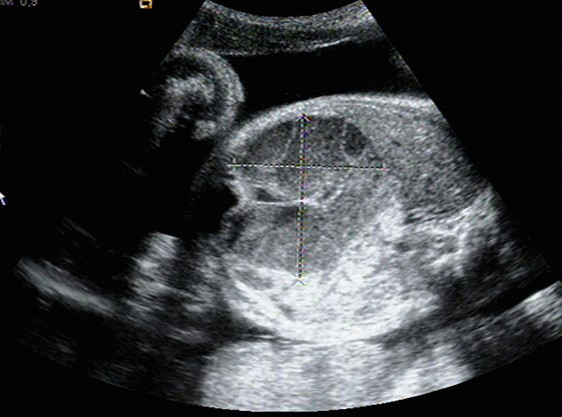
Ultrasound image: 32.3 weeks of gestation. Transverse scan image thorough the fetal abdomen identifying a mass occupying the entire left hemiabdomen (meconium pseudocyst), with mixed echogenicity. No calcifications were observed.
